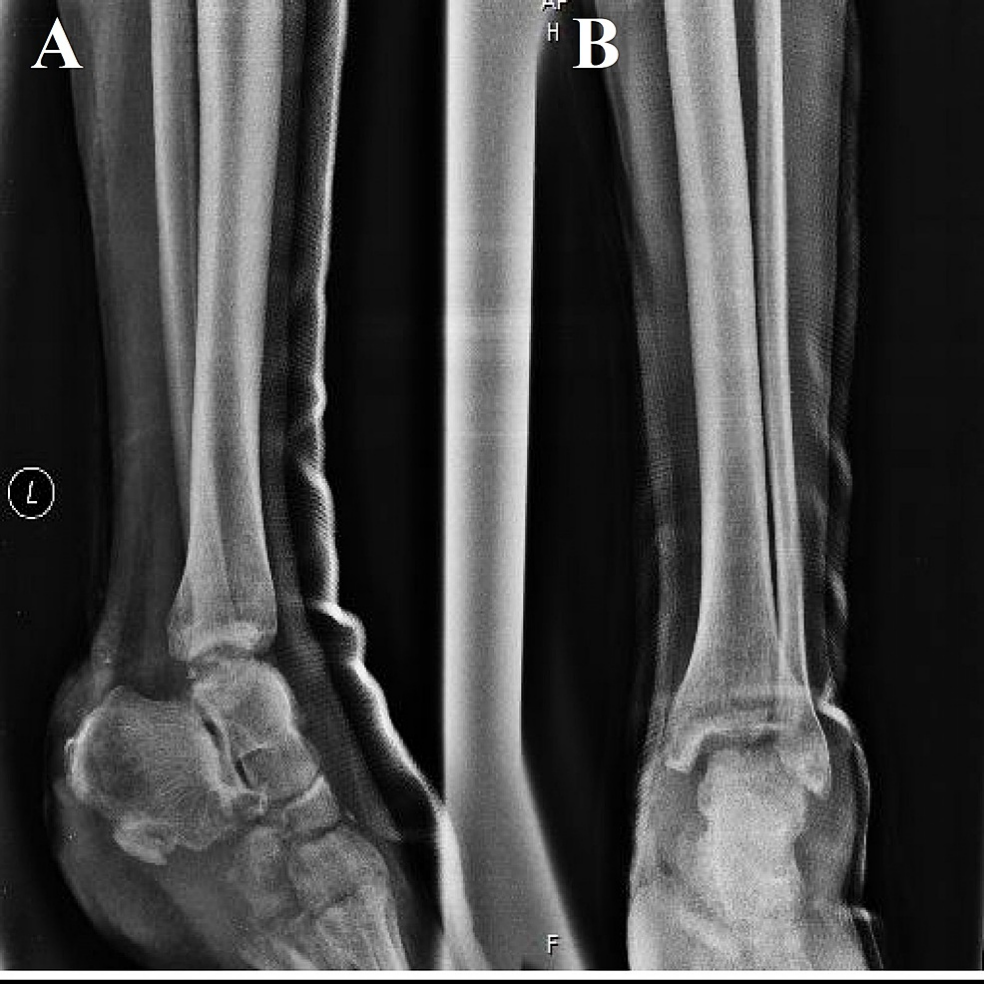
Anteroposterior (A) and lateral (B) radiograph displaying the avulsion fracture of the calcaneus and the fractures in the cuboid and cuneiform bones.
